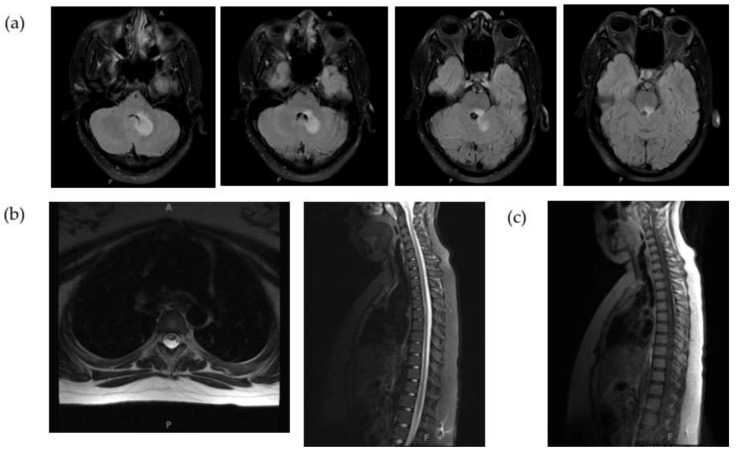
Brain and spine MRI of patient at initial demyelinating event. (a) T2 hyperintense lesion predominantly located within the white matter of the left cerebellar hemisphere extending to the brachium pontis and posterior brainstem. (b) Intramedullary T2 hyperintense lesion along the right aspect of the cord at T4–T5. (c) Mild enhancement of spine lesion.
